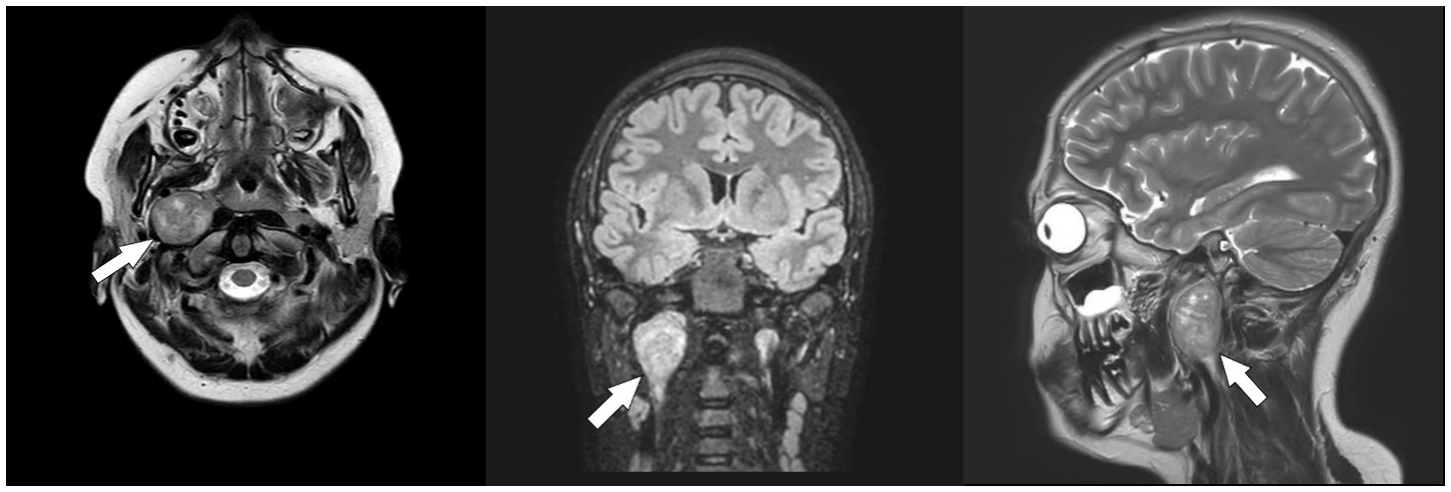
Pre-operative Magnetic Resonance Imaging (MRI). Axial T2-weighted (*left*), coronal fluid-attenuated inversion recovery (FLAIR) (*middle*), and sagittal T2-weighted (*right*) scans showing a neoformation arising from the hypoglossal nerve (*white arrows*).
